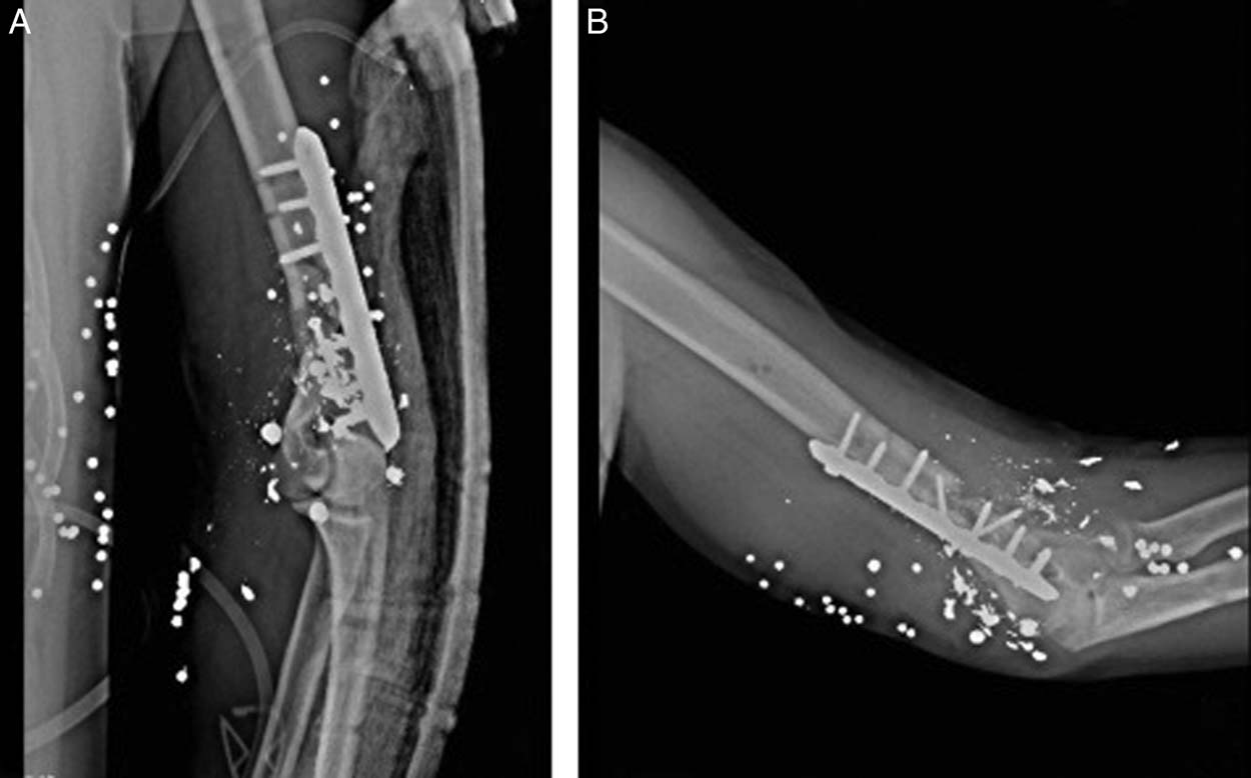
(A) Postoperative X-ray, after open reduction and internal fixation with allograft and splint fixation. (B) It is failure 6 months later.
